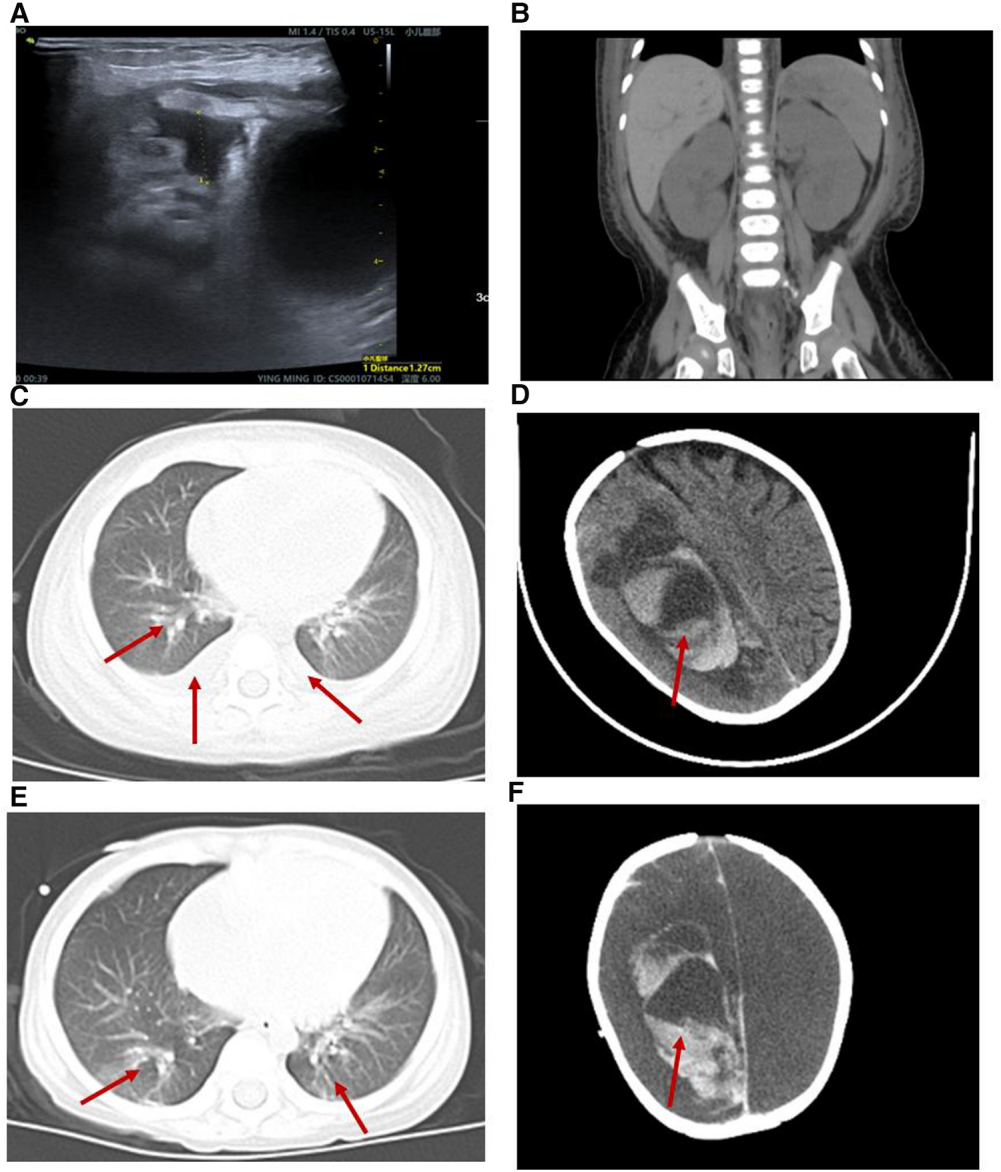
Radiological alterations during the patient's hospitalization. (A) B-ultrasound showed a small amount of abdominal effusion. (B) Abdominal computed tomography (CT) showed that the kidney, liver and spleen increased in volume with a low-density image. (C,E) Chest CT displayed both lung pneumonia and partial atelectasis of the upper lobe of the right lung. (D) Head CT showed that the subarachnoid hemorrhage during the 6th week of his hospital stay. (F) Head CT showed that the subarachnoid hemorrhage was more extensive during the 8th week of his hospital stay.
